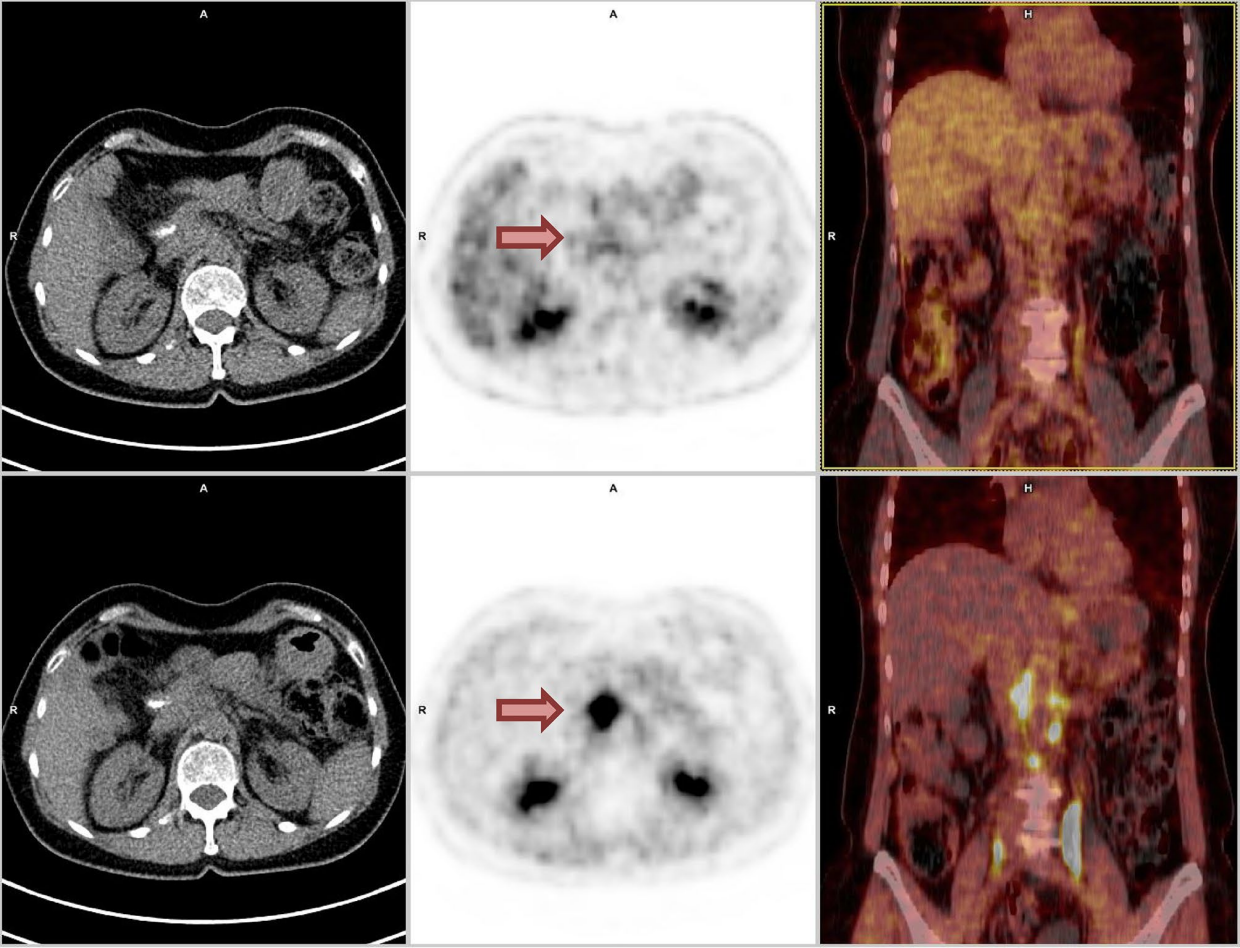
Showing patient H with a lymphonodular metastatic pancreatic ductal adenocarcinoma at timepoint *t*_*1*_. Upper and lower left-side: unenhanced CT; upper middle column: [^18^F]FDG-PET; lower middle column: [^68^Ga]FAPI-PET (both axial reconstructions at the level of the kidneys); upper and lower right-side: fused mode [^18^F]FDG-PET/CT (upper row) and [^68^Ga]FAPI-PET/CT (lower row) displaying assessment of index lesions (red arrows) [point-spread function (PSF) reconstruction; PET Heat 2D, 55 min after 101 MBq [^18^F]FDG injection and 27 min after 106 MBq [^68^Ga]FAPI-injection]. Uptake on [^18^F]FDG-PET-images [^18^F]FDG-uptake was minimal and only slightly elevated compared to background noise.
